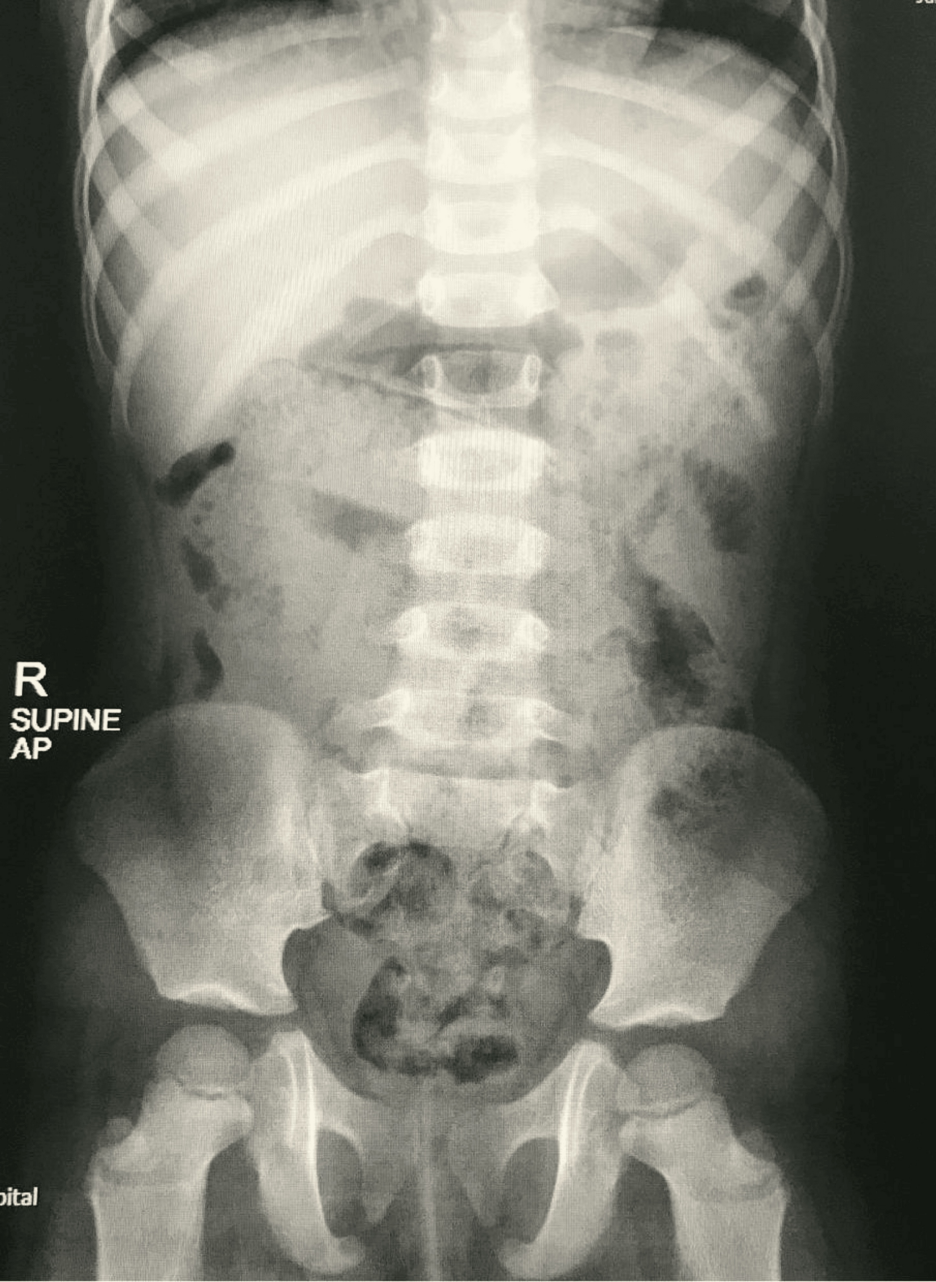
On readmission, 72 hours later, abdominal X-ray (supine) shows fecalization of the small bowel.
